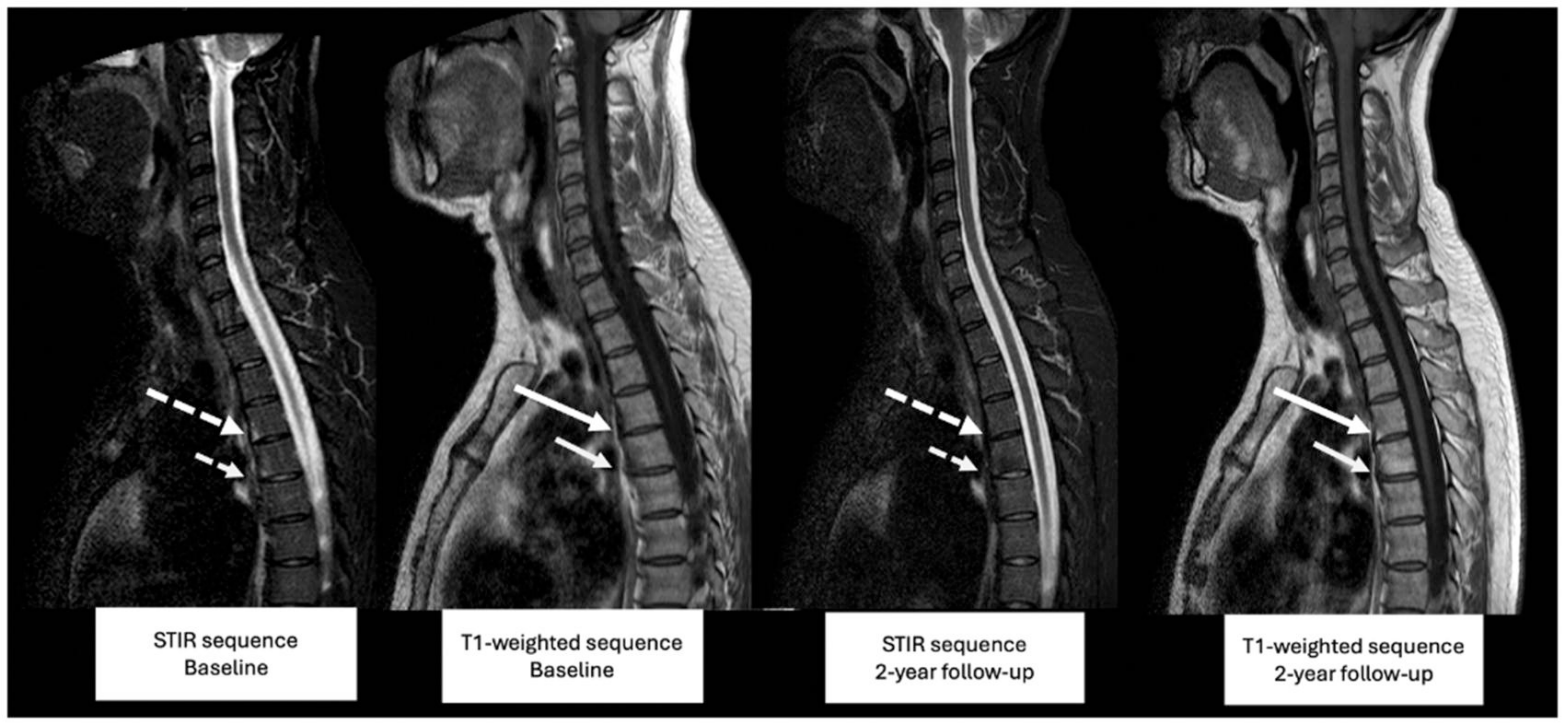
MRI lesion progression in axSpA: from bone marrow oedema to fat lesion or no change. Bone marrow oedema (BME) in the lower anterior quadrant of T4 at baseline (large, dashed arrow, STIR) without a corresponding structural lesion on T1-weighted imaging (large, solid arrow) progressed to a fat lesion after 2 years (large, solid arrow, T1-weighted), without corresponding inflammation (large, dashed arrow, STIR, 2-year). BME in the lower anterior quadrant of T5 at baseline (small, dashed arrow, STIR) without a corresponding structural lesion (small, solid arrow, T1-weighted) showed no new fat lesion after 2 years (small, solid arrow, T1-weighted) and no inflammation (small, dashed arrow, STIR, 2-year). Lesions were present on more than one consecutive slice
